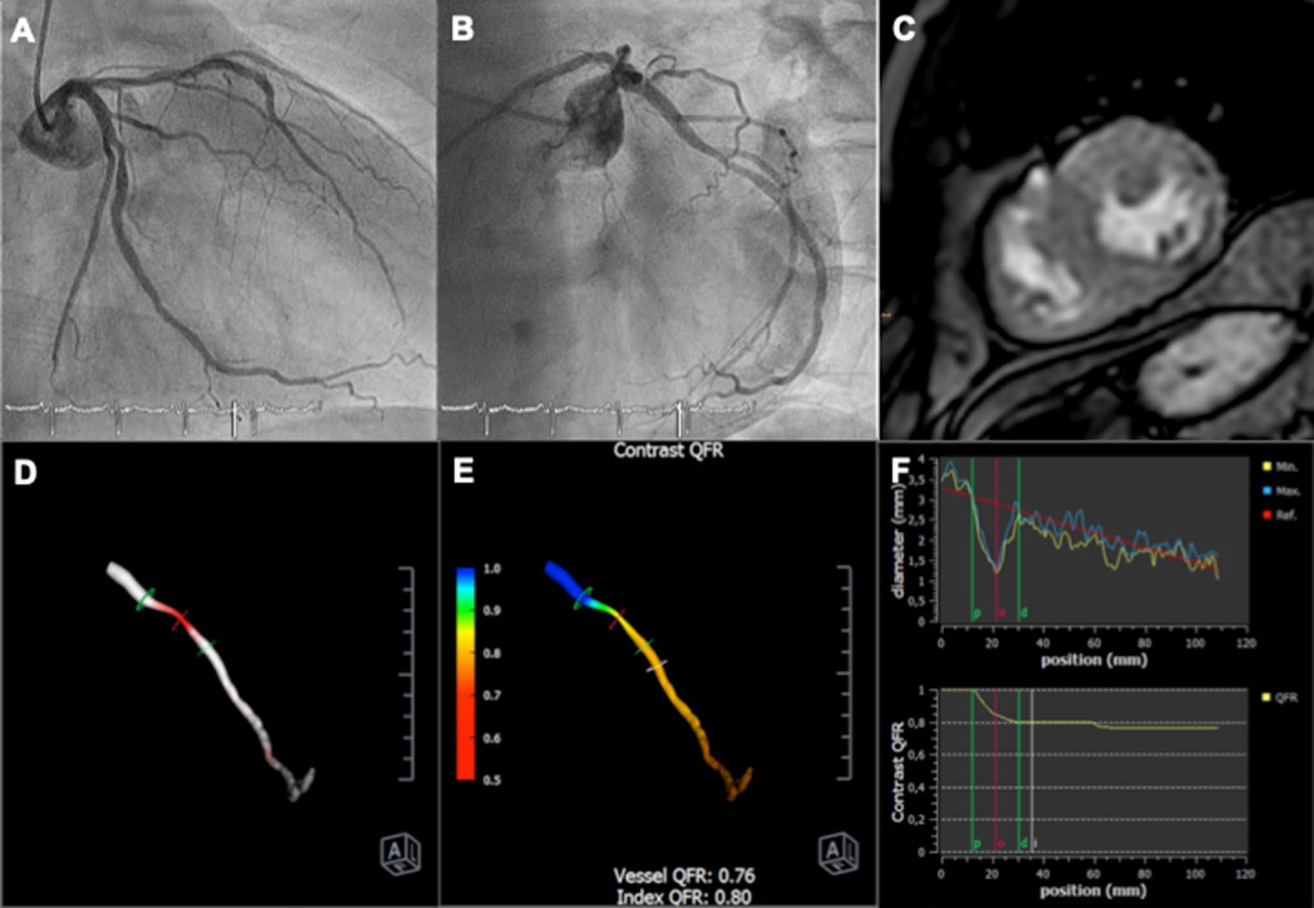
Representative examples. a Right anterior oblique (RAO) 30° projection of the left coronary artery. b, Coronary angiogram of the left coronary artery in left anterior oblique (LAO) 60° angulation. c, Stress MRI showing a perfusion deficit in the midventricular inferolateral segment of the left ventricle. d, 3D-Reconstruction of the dominant 2nd marginal branch in LAO 28° and 24° caudal projection. e, 3D Reconstruction and visualisation of the dominant 2nd marginal branch with QFR. Vessel QFR characterises the whole vessel and index QFR pointes out the QFR at the white marker. f, Lumen dimensions showing minimal, maximal and reference diameters (above) and virtual cQFR pullback (below) with p representing the proximal lesion marker, d the distal lesion marker and o the lesion centre
